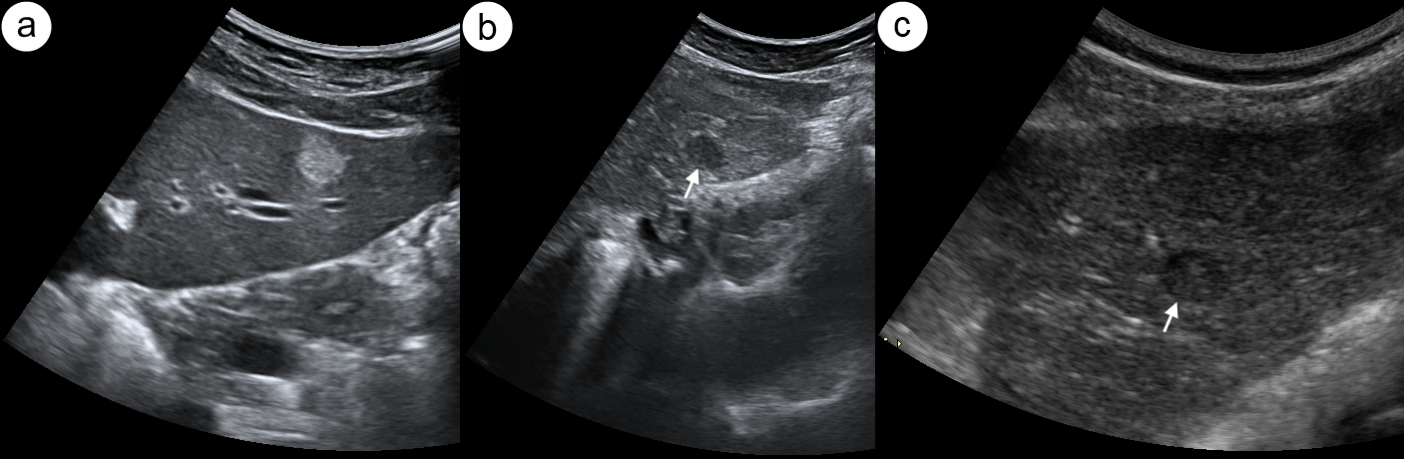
Hepatic hemangioma in US. Transverse sonogram of left lobe of liver presents typical hyperechogenic hemangioma (a). Figure 1b and c show two different hypoechogenic liver lesions suspected to be atypical hemangioma, subsequently confirmed in CT.
